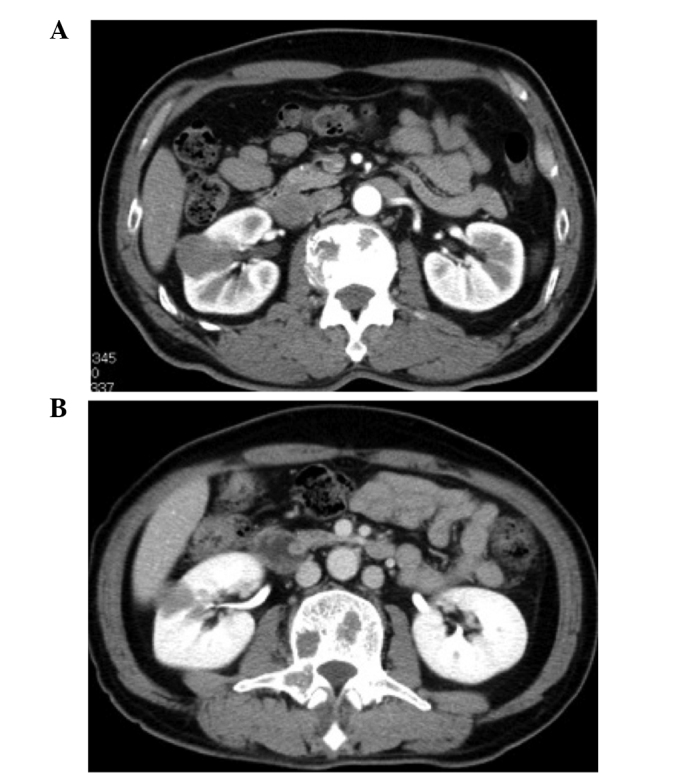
(A) Pretreatment CT revealed a right renal tumor measuring 3.5 cm in diameter. (B) Post-treatment CT demonstrated tumor shrinkage in the right kidney following combination chemotherapy with gemcitabine and doxorubicin. CT, computed tomography.
